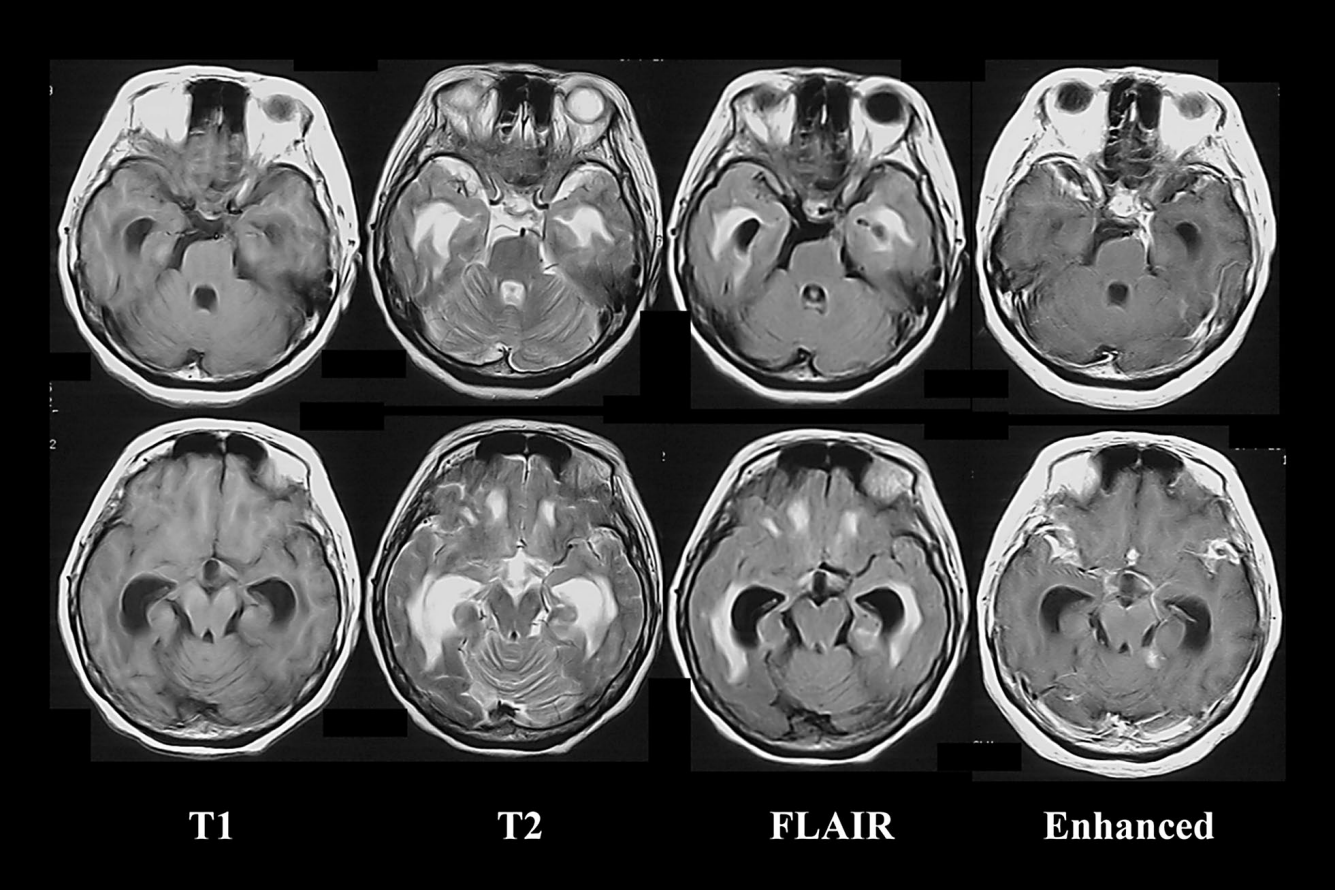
Brain magnetic resonance imaging on admission. The scan revealed hydrocephalus and an abnormal enhancement effect at the brain base
